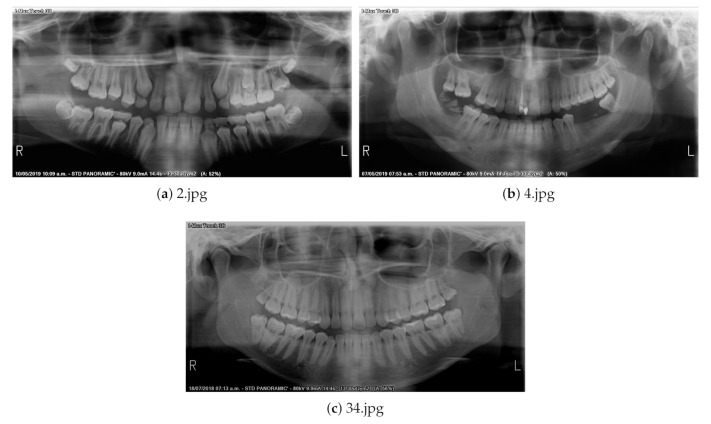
Example panoramic radiographs: (a) mixed dentition; (b) partially edentulous permanent dentition; and (c) complete permanent dentition.
